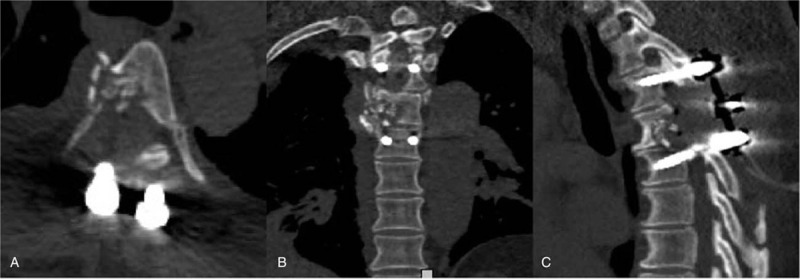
Postoperative CT images. Postoperative axial (A), coronal (B), and sagittal (C) CT imaging have been obtained with the bone window, showing fragments of bone from T5 and instrumented spinal stabilization on the 7th day after surgery.
